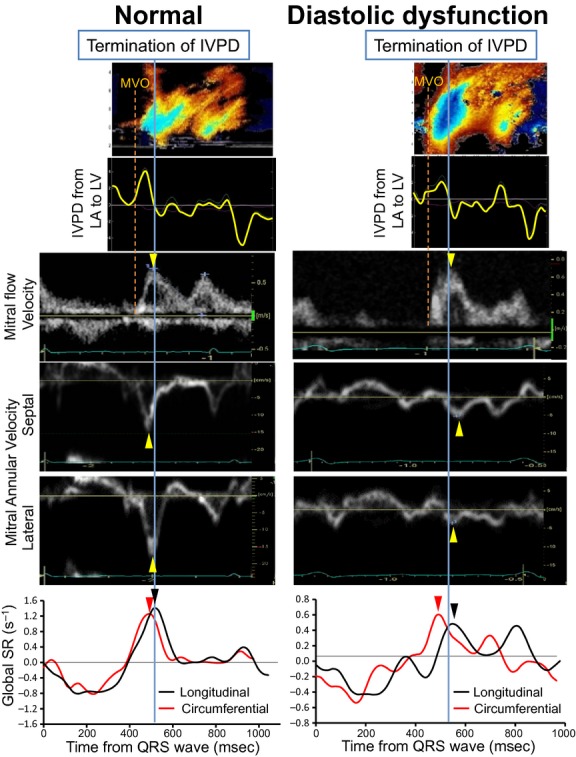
Color M‐mode Doppler image, IVPD from LA to LV, mitral flow velocity, global longitudinal SR curve, and global circumferential SR obtained from a patient with normal diastolic function (Normal) (left) and a patient with restrictive filling (Diastolic Dysfunction) (right). In the normal, the peak e′ and longitudinal and circumferential SR_E_ occur coincident with or prior to the peak mitral flow velocity (E) and the termination of the IVPD. In contrast, in diastolic dysfunction, e′ and longitudinal SR_E_ were delayed occurring after E and after the termination of the IVPD whereas E and the circumferential SR_E_ were not delayed. Abbreviations are the same as Figure 2.
